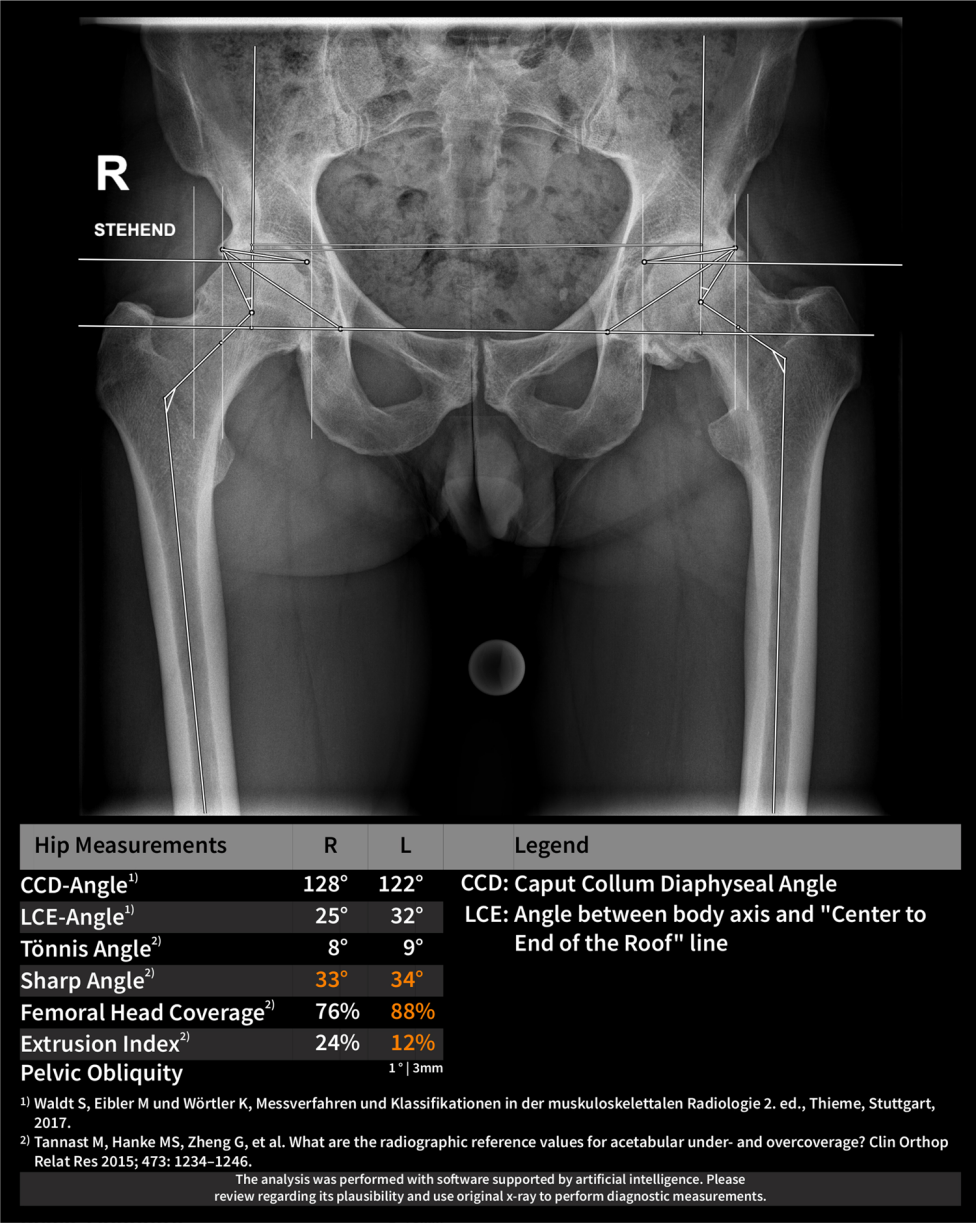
Example output of the AI software for the evaluation of pelvic radiographs
